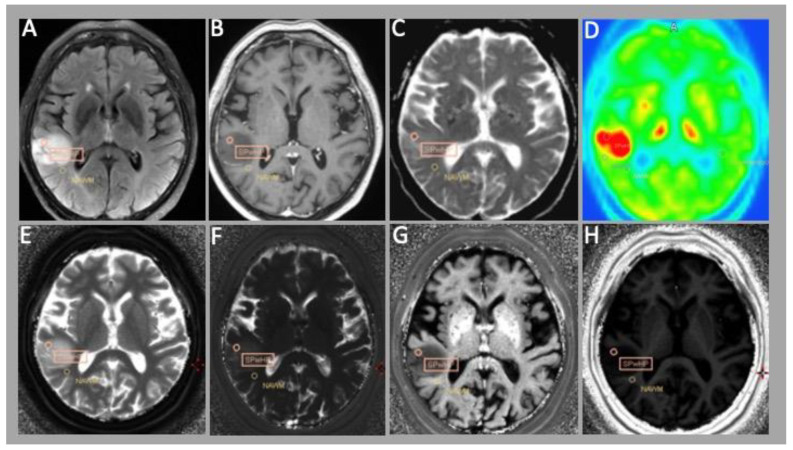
Patient with an astrocytoma Gr. 3 according to WHO 2021 classification with MET PET-positive tumor. From upper left to lower right: conventional MRI sequences—(A) FLAIR (Fluid attenuated inverse recovery); (B) T1w images with contrast enhancement; (C) ADC map; and (D) methionine PET image. MRF images—(E) T1 map; (F) T2 map; (G) R2 map; and (H) R1 map.
